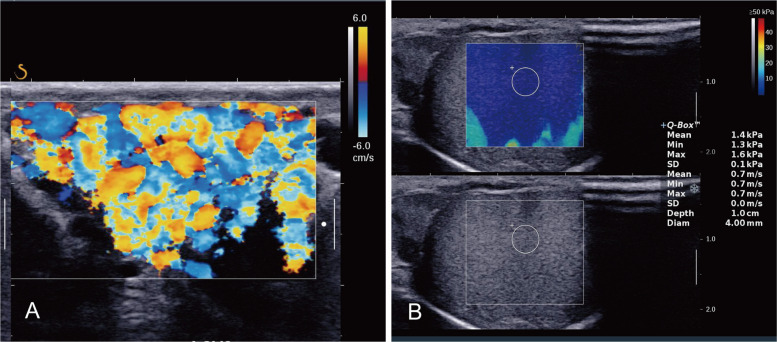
A 28–year–old man diagnosed with grade III left varicocele. A: Color doppler flow imaging of the left spermatic vein. B: SWE value of the left testis was 1.4 kPa, measured using the SL15–4 probe with a 4 mm ROI
